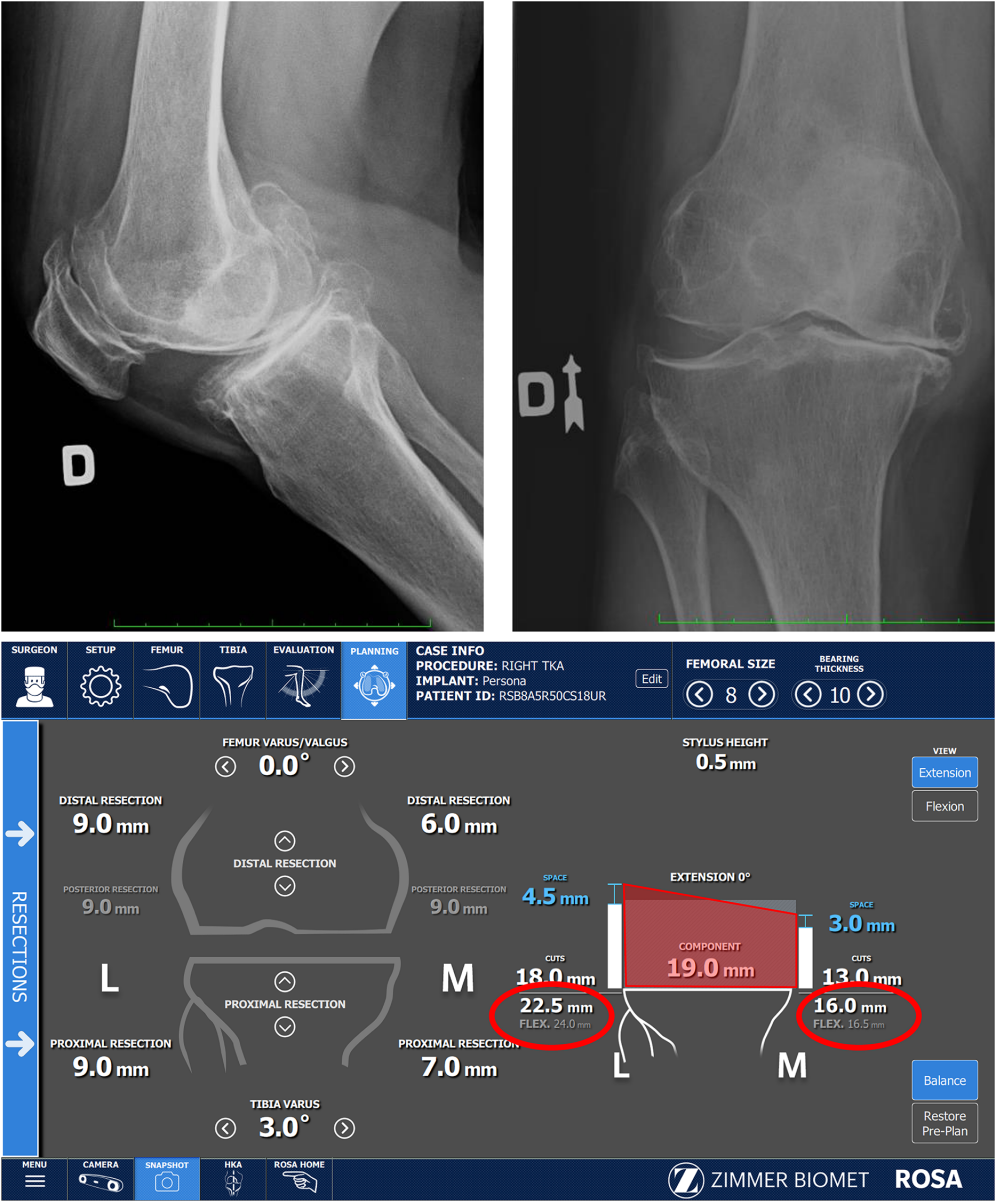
Large posterior osteophyte present in x-ray, explaining flexion deformity. Medial osteophytes still present, as seen in the x-Ray, are reflected on the registration screen as inadequate space in the medial compartment (16 mm of space compared to 19 mm component thickness).
